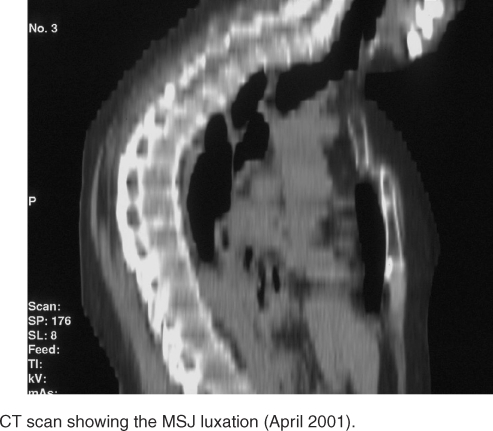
CT scan showing the MSJ luxation (April 2001).
